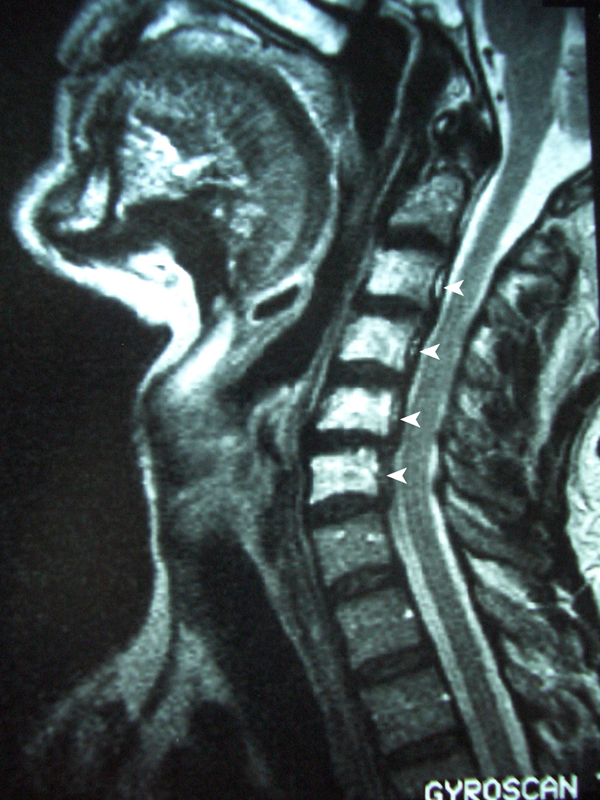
December 1997: MRI scan of the neck showed marked narrowing of the airway. An enhanced signal in C2 to C6 vertebral bodies (white arrows) and a loss of height in C5 were noted and deemed consistent with post-radiotherapy change.
